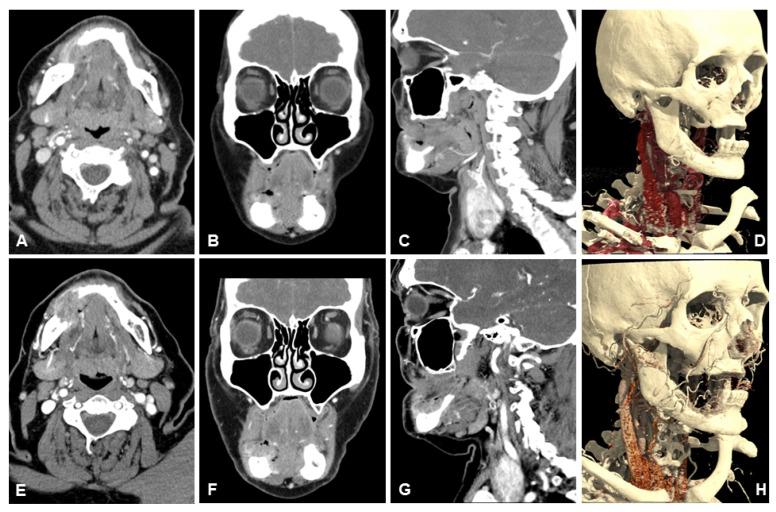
Example of a 79-year old male patient with a squamous cell carcinoma of the right lower jaw. Patient initially refused surgery, thus a 3-months follow-up examination was performed. Note the clear depiction and hypervascularization of the tumor margins and the lower noise of UHR-CT, especially in the axial plane. In the coronal reconstruction you can beautifully depict the tumor infiltration of the perimandibular fat and the contours of the sublingual space. (A) axial 3 mm reconstruction NR-CT, (B) sagittal 3 mm reconstruction NR-CT, (C) coronal 3 mm reconstruction NR-CT, (D) best possible 3D-reconstruction NR-CT, (E) axial 3 mm reconstruction UHR-CT, (F) sagittal 3 mm reconstruction UHR-CT, (G) coronal 3 mm reconstruction UHR-CT, and (H) best possible 3D-reconstruction UHR-CT.
